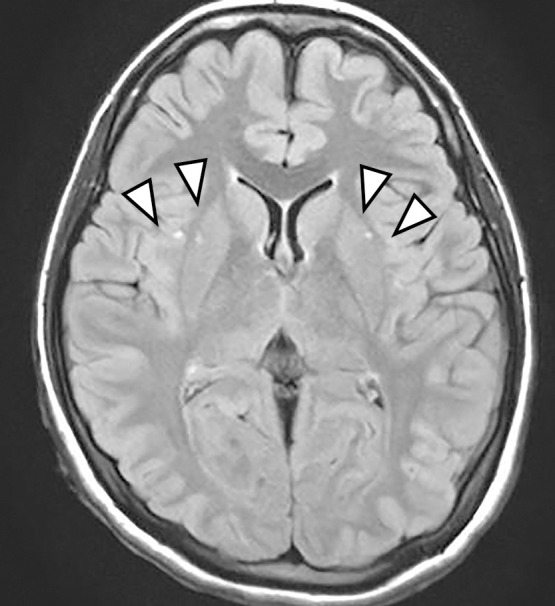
Post-treatment head MRI axial section with fluid-attenuated inversion recovery. High-intensity areas appeared in the bilateral lateral putamina and mild high-intensity areas were observed in the hippocampal limbic system (arrows), which are consistent with findings of anti-NMDA receptor encephalitis.
